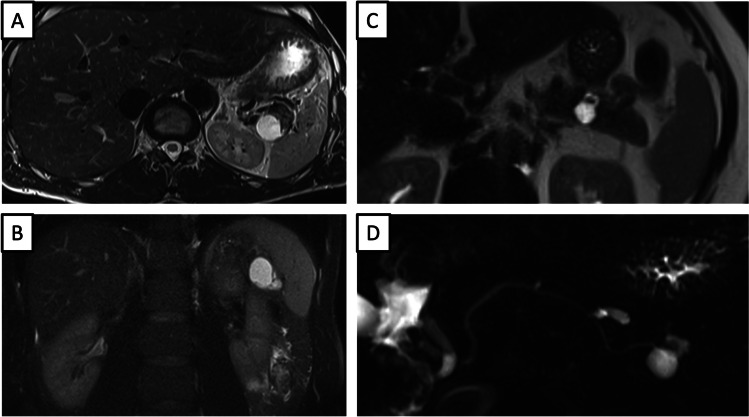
Comparison of lymphoepithelial cyst (LEC) and branch-duct intraductal papillary mucinous neoplasm (BD-IPMN). (a, b) A 44-year-old male patient with a cystic lesion within the pancreatic tail. Axial T2-weighted MR image without fat suppression (a) shows LEC in the distal part of the pancreatic tail with a similar image to a BD-IPMN. Coronal T2-weighted MR image with fat suppression (b) demonstrates the septations of the lesion. (c, d) A 48-year-old male patient with BD-IPMN in the pancreatic tail. Axial T2-weighted MR image without fat suppression (c) shows a side branch IPMN in the pancreatic tail. Coronal MRCP MR image (d) shows the communication of the lesion to the main pancreatic duct, which was the key differential feature
